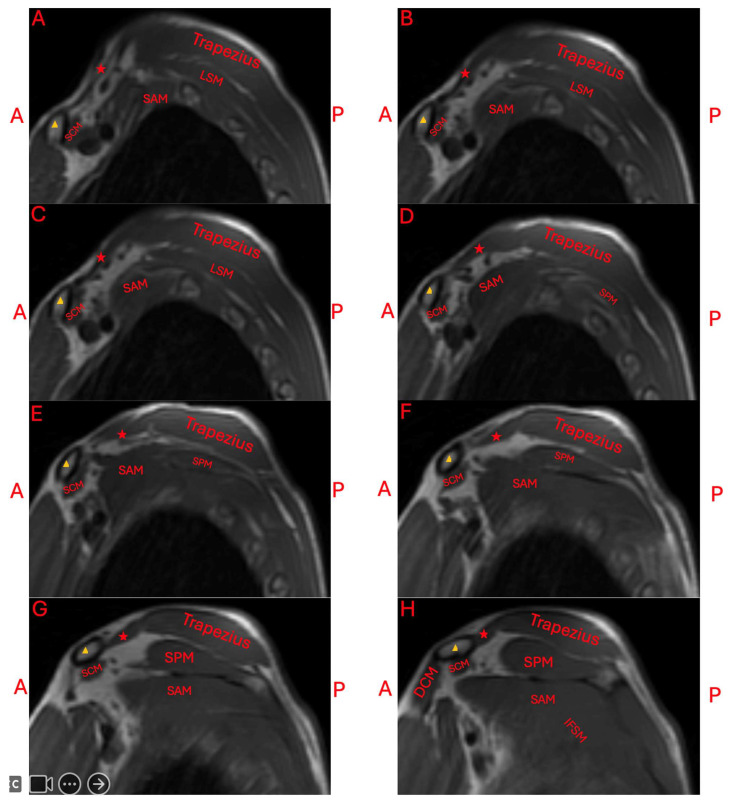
Sagittal T1-weighted MRI imaging of the right shoulder. Progression from the medial to lateral shoulder view can be followed from (A–H). The images are oriented anteriorly on the left and posteriorly on the right. Red stars mark the levator claviculae muscle, and yellow triangles mark the clavicle. Other muscles include the levator scapulae muscle (LSM), serratus anterior muscle (SAM), subclavius muscle (SCM), supraspinatus muscle (SPM), infraspinatus muscle (IFSM), and deltoid clavicular part (DCM).
